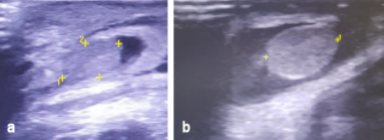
a) Ultrasonography of the right testicle. b) Ultrasonography of the left testicle. Both testicles are depicted inside the scrotum. The scrotal wall as well as the testicles are grossly thickened and edematous, while the echogenic structure and vascularity of the testicles are normal.
